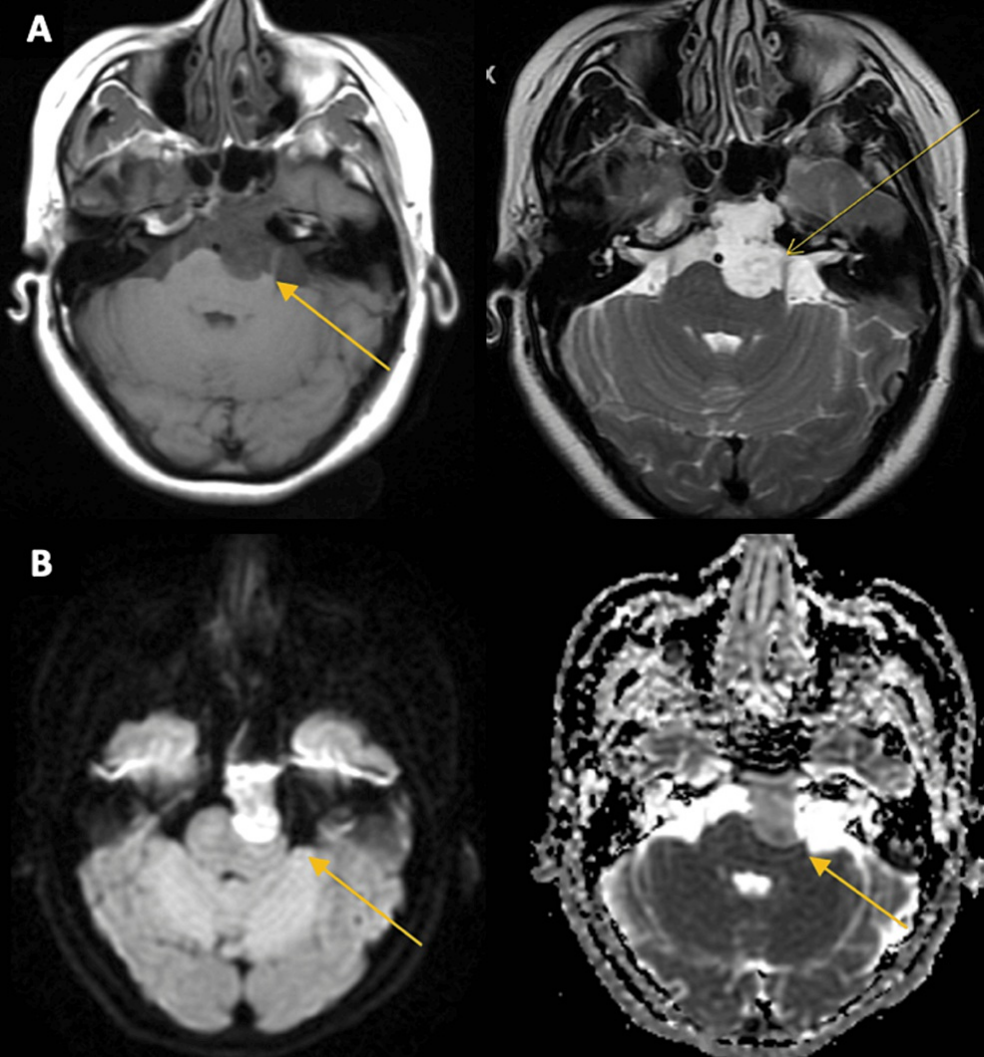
An MRI of the brain demonstrates a lesion of the prepontine cistern with a mass effect on the left pons and fourth ventricle, with restricted diffusion and small amounts of internal enhancement. A: T1 imaging, pre-contrast, and post-contrast B: diffusion-weighted imaging and diffusion coefficient imaging
